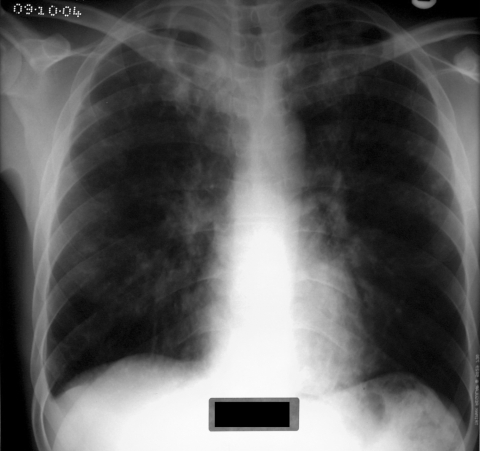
Chest radiograph of a tuberculosis patient addicted to crack cocaine.
